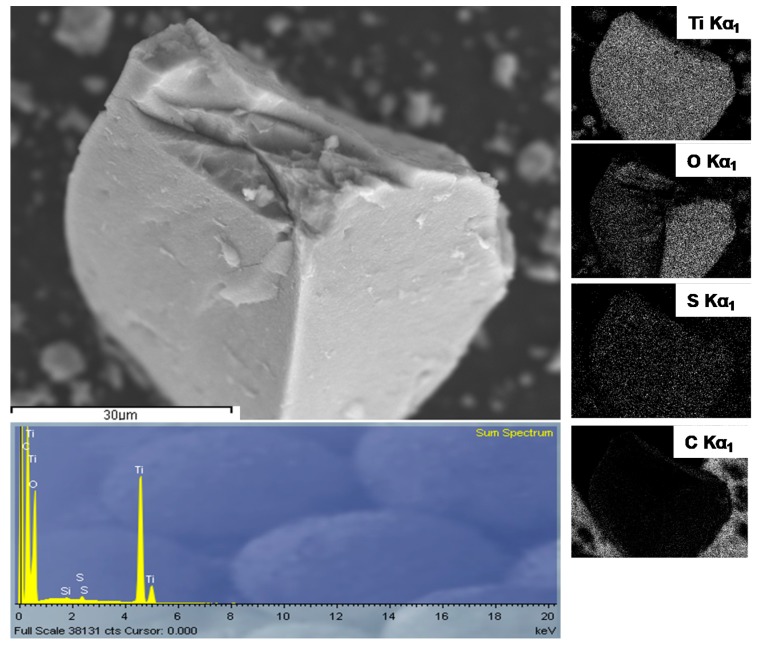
Energy dispersive X-ray (EDX) analysis of S-TiO_2_/rGO(5.0%).
